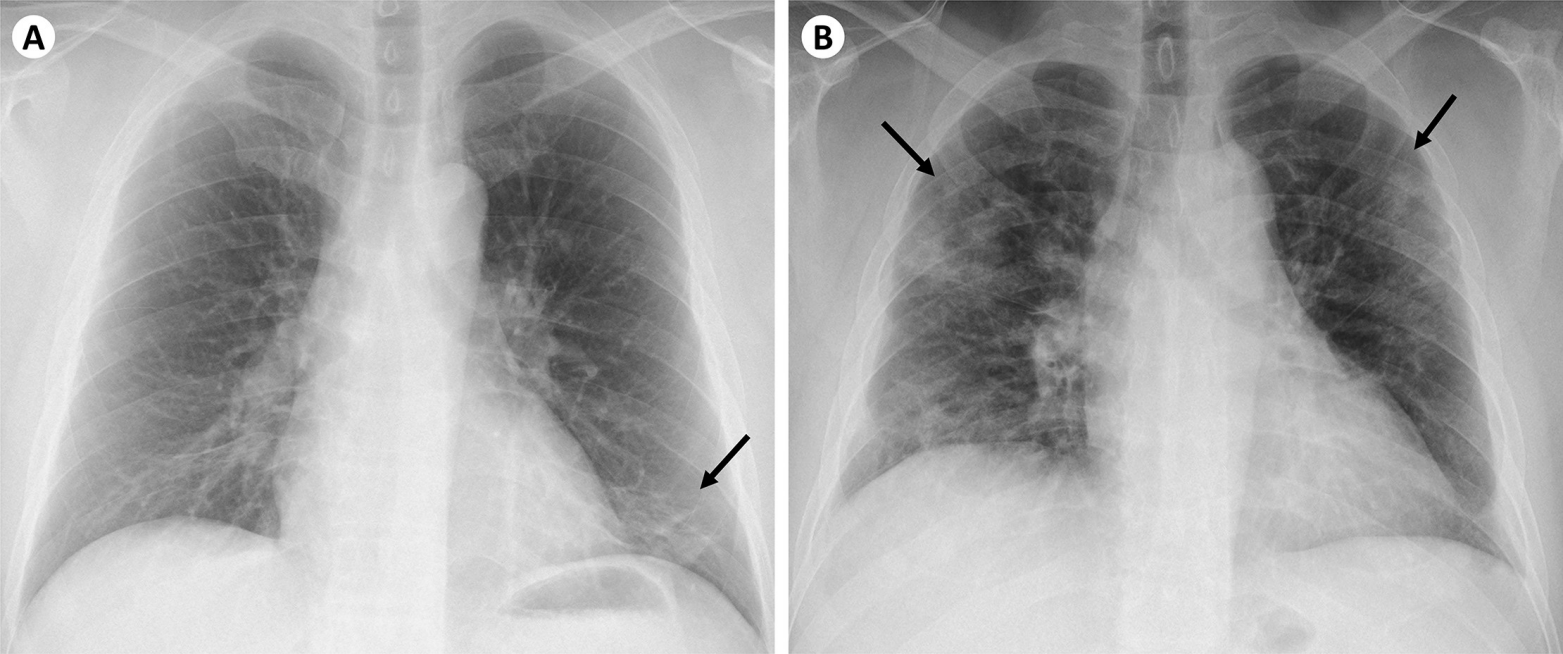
Example radiographs of (A) low-risk CXR and (B) high-risk CXR. (A) Low-risk CXR showing unilateral opacity (demarcated by black arrow) in the left lower zone. This patient’s symptoms resolved after 3 days and he did not require supplemental oxygen throughout his admission. (B) High-risk CXR showing bilateral, multifocal opacities involving the upper zones (demarcated by black arrows). This patient developed hypoxia requiring supplemental oxygen during his admission.
